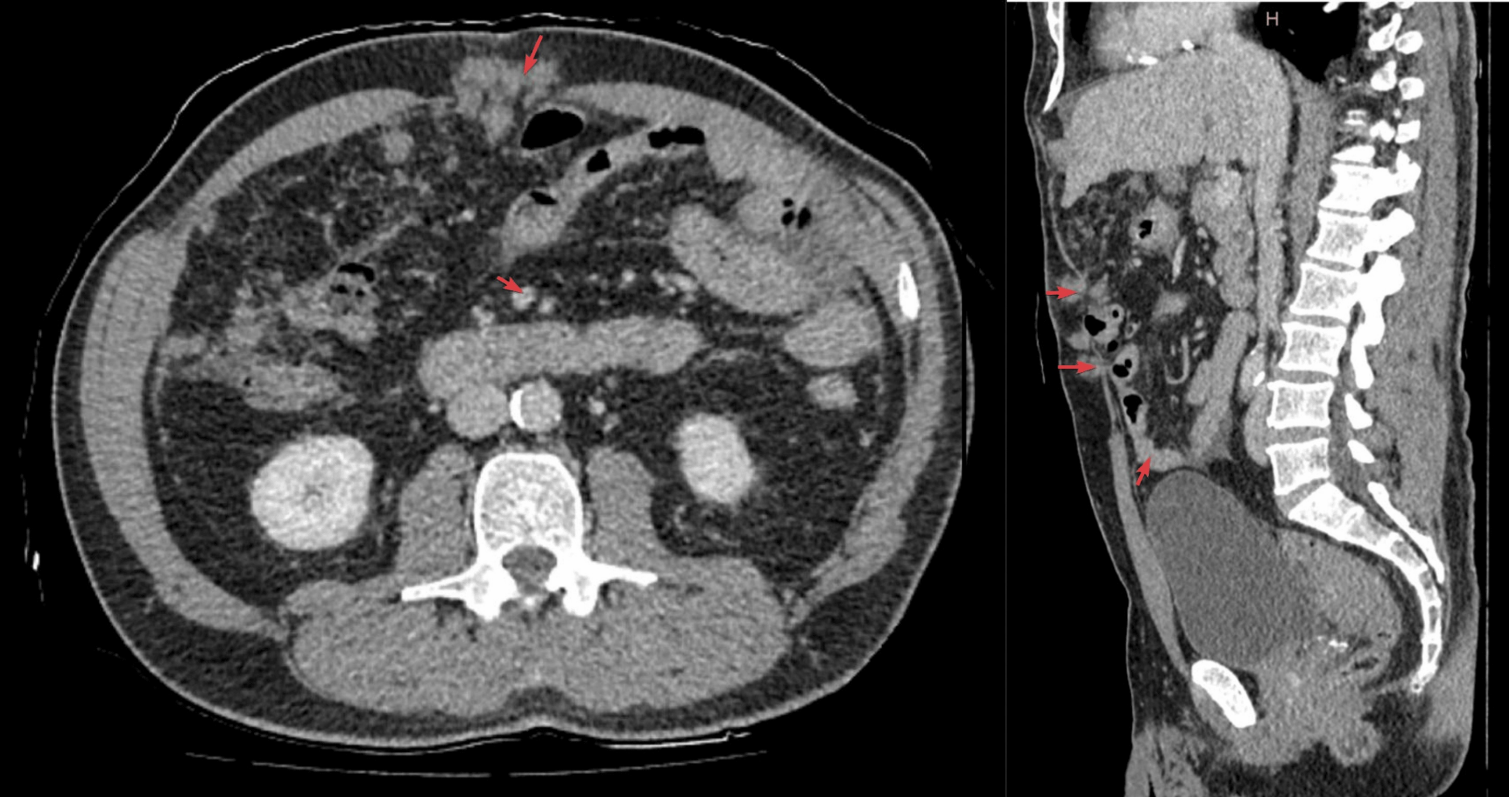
CT abdomen and pelvis with contrast (May 4, 2024) demonstrating extensive omental and peritoneal deposits. The arrows indicate areas of omental and peritoneal deposits.
